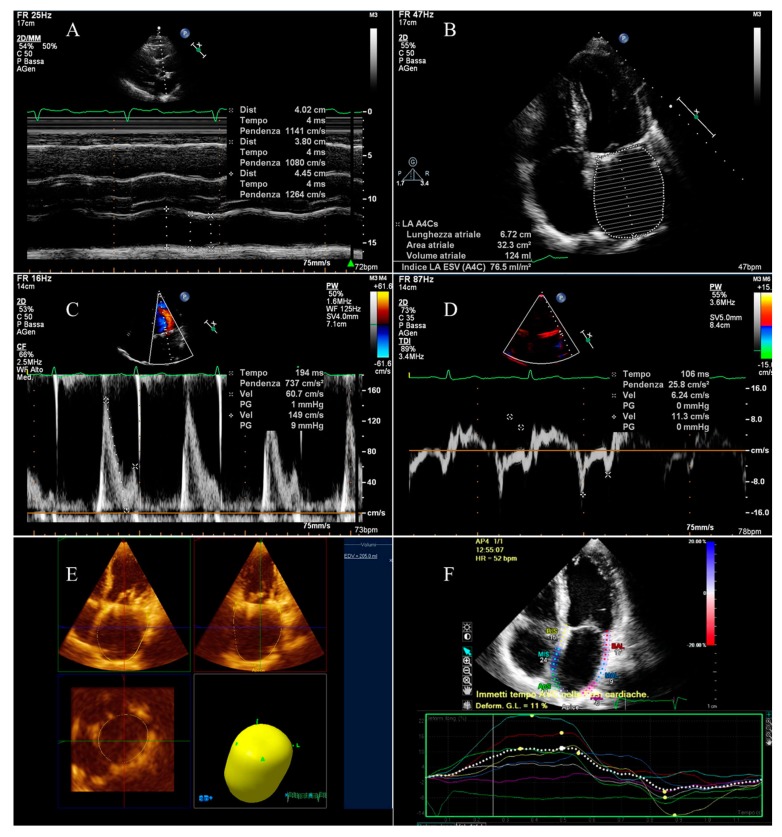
Echocardiographic evaluation of left atrial function: (A) measurement of antero-posterior diameters; (B) evaluations of areas and volumes and measurement of maximum supero-inferior diameter; (C) measurement of transmitral peak velocity of the late filling wave (A wave); (D) measurement of lateral mitral annulus peak velocity related to atrial contraction (a’ wave) and evaluation of atrial conduction delay; (E) calculation of maximum 3D volume; (F) evaluation of global longitudinal atrial strain.
